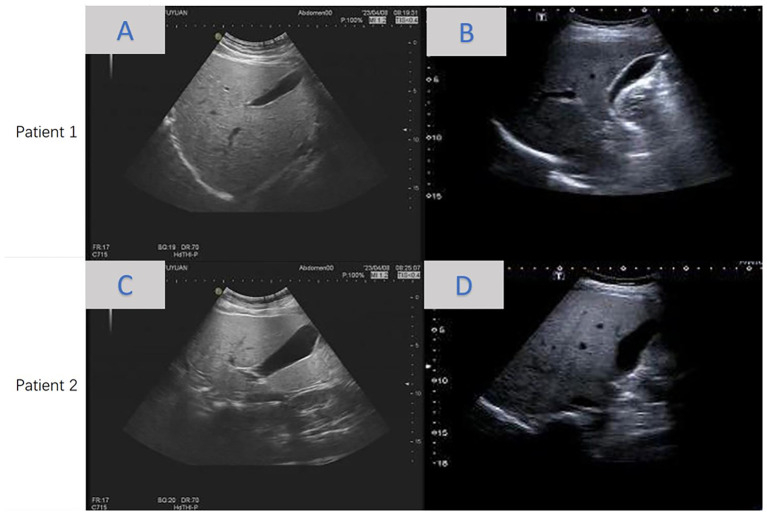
Abdominal ultrasound images of Patient 1 (A, B) and Patient 2 (C, D) at baseline (A, C) and after 11 months of treatment (B, D).
